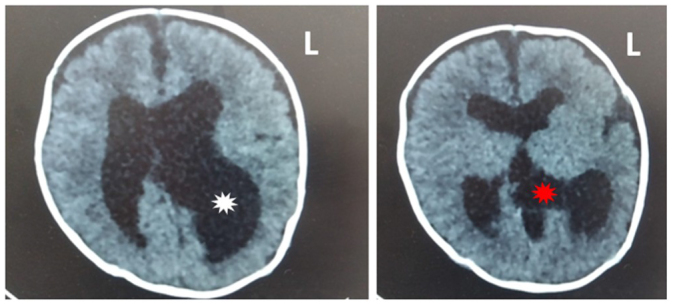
A brain CT scan showing asymmetric hydrocephalus. The left image highlights a more pronounced dilation of the left lateral ventricle (white asterisk) than the right. The right image demonstrates a dilated third ventricle (red asterisk). CT, computed tomography.
